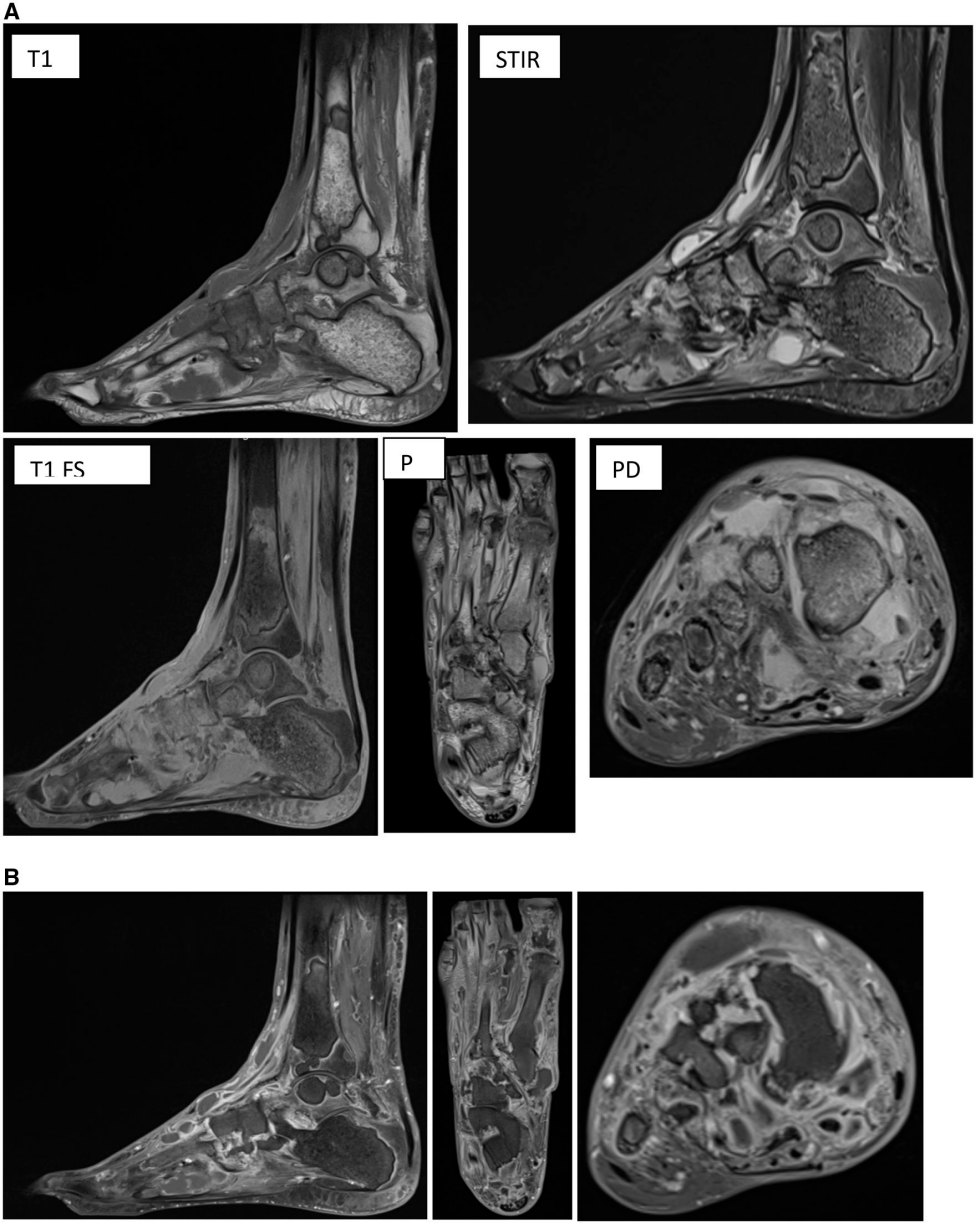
Multi-sequence MRI images of the right ankle demonstrating subcutaneous panniculitis and intramedullary fat necrosis. T1 FS post-gadolinium contrast in sagittal, long and coronal (left to right). (A) T1 fat saturated and STIR images demonstrate innumerable intramedullary lesions throughout the bones of the right lower limb. They have a serpiginous border with a double rim (on STIR sequence there is a hyperintense line paralleling the inner surface of the hypointense line within the marrow). Internally the lesions demonstrate T1 hypointensity with mild heterogenous T2 hyperintensity. (B) T1 post-gadolinium contrast images demonstrate mild peripheral contrast enhancement with adjacent bone oedema.
